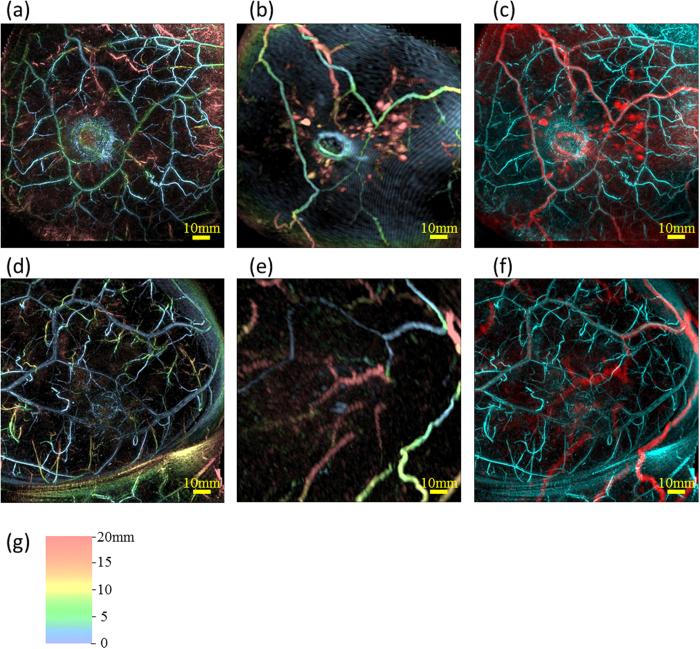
Examples of breast deformation of MRI and comparison of visibilities of blood vessels between PAI and MRI using maximum intensity projection (MIP) images on the healthy breast. Case 1 (Fig. 1(a–c)): (a) PA image, (b) MR image deformed to correspond to the PA image, and (c) fusion image of PA (cyan) and MR (red). Case 2 (Fig. 1(d–f)): (d) PA image, (e) MR image deformed to correspond to the PA image, and (f) fusion image of PA (cyan) and MR (red). All images are coronal views. In Fig. 1(a), (b), (d) and (e), we colored the signals according to the depth using the color chart shown in Fig. 1(g). This color chart for depth information is also used in Figs 2, 4 and 5.
